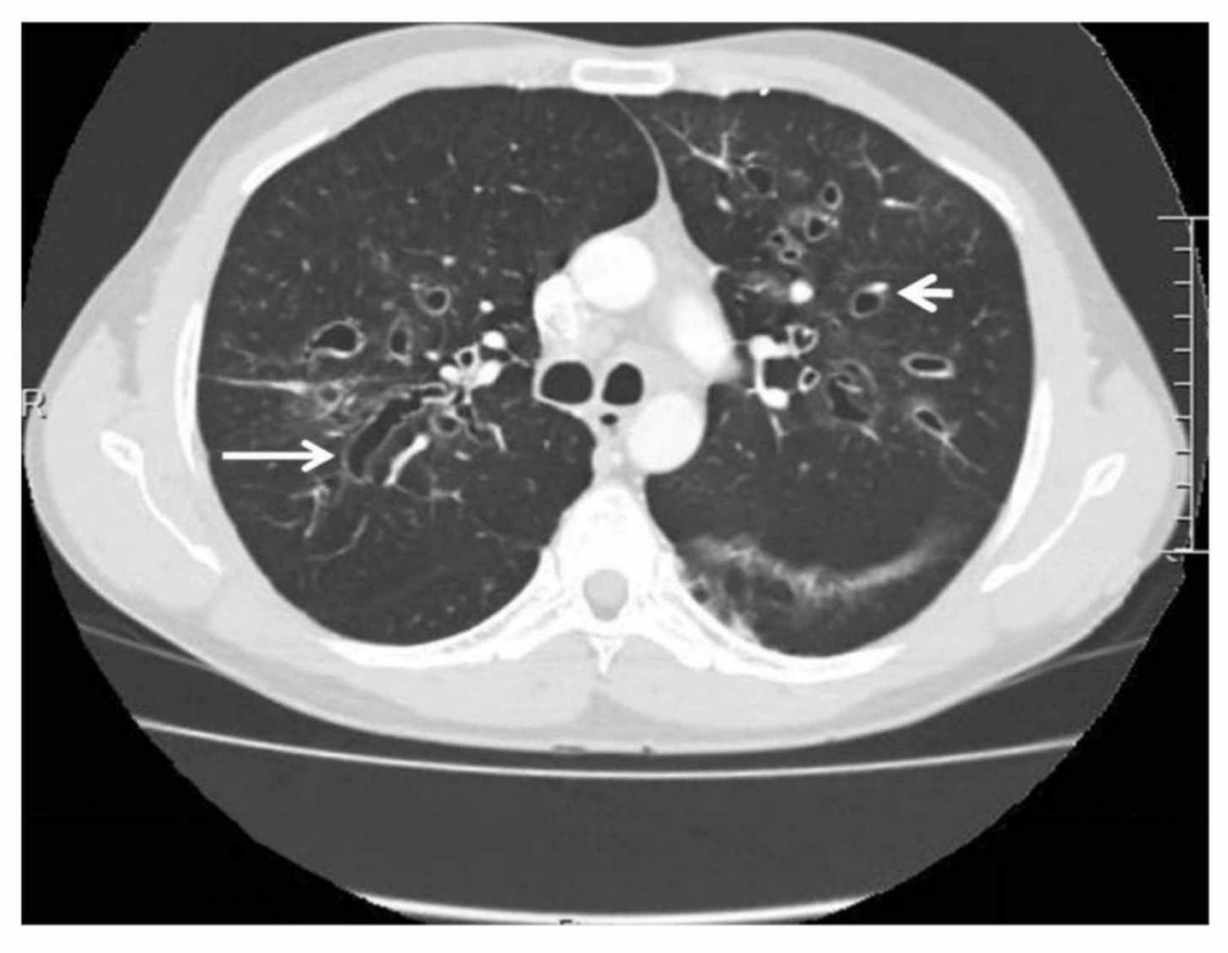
Computed Tomography Scan of Thorax Showing Central Bronchiectasis in Case of ABPA This is a computed tomography (CT) scan of the thorax showing central bronchiectasis [11]. It is identified by the 'signet ring' (short, thick arrow) and 'string of pearls' (long, thin arrow) appearances [11]. Mucoid impaction and dilated bronchi are also present [11].
